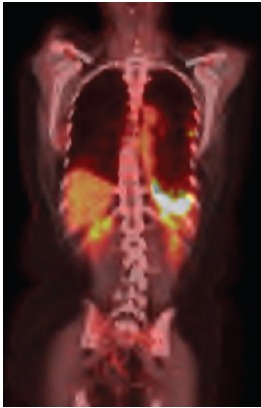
PET–CT after second–line therapy revealing radiopharmaceutical captation maintanence.
